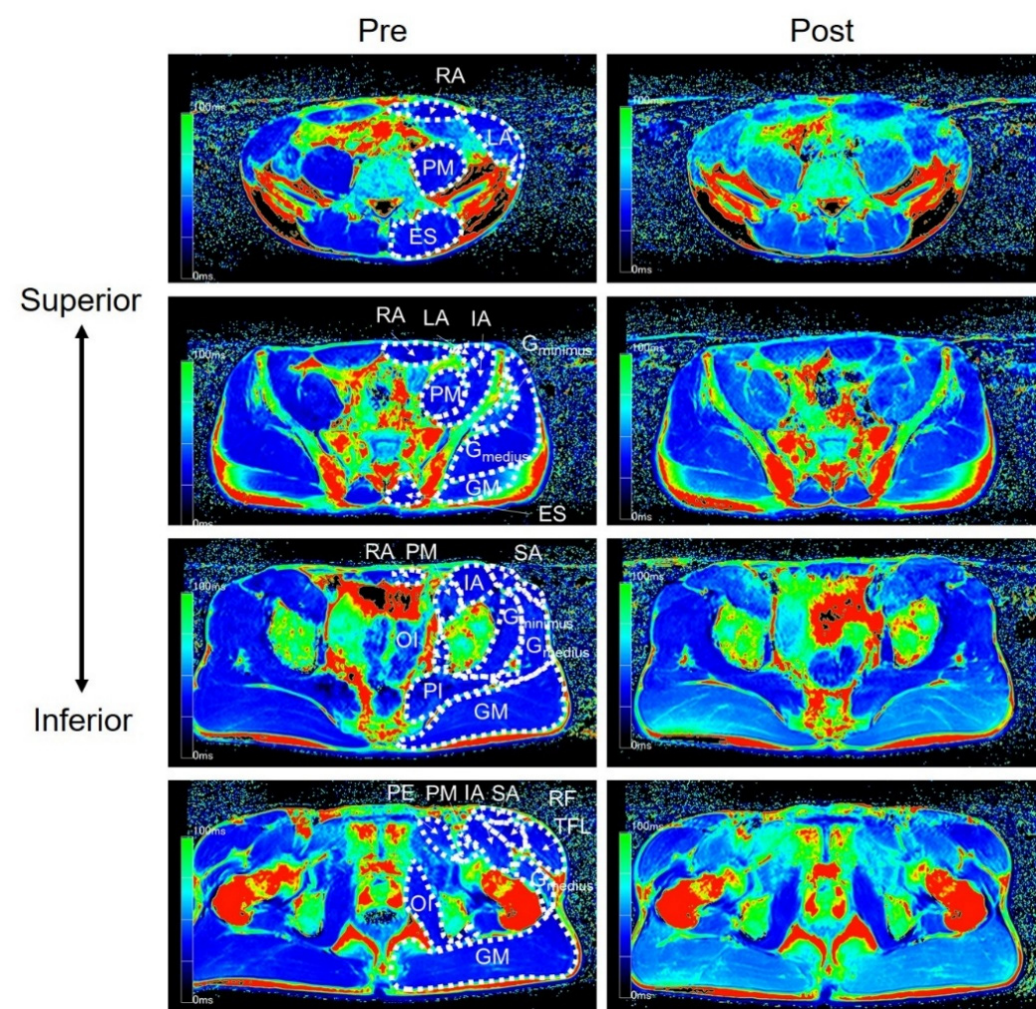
Examples of T2-weighted magnetic resonance images of the trunk and the pelvis. RA, rectus abdominis; LA, lateral abdominals; PM, psoas major; ES, erector spinae; IL, iliac; GM, gluteus maximus; G_medius_, gluteus medius; G_minimus_, gluteus minimus; PI, piriformis; SA, sartorius; TFL, tensor fasciae latae; RF, rectus femoris; PE, pectineus
